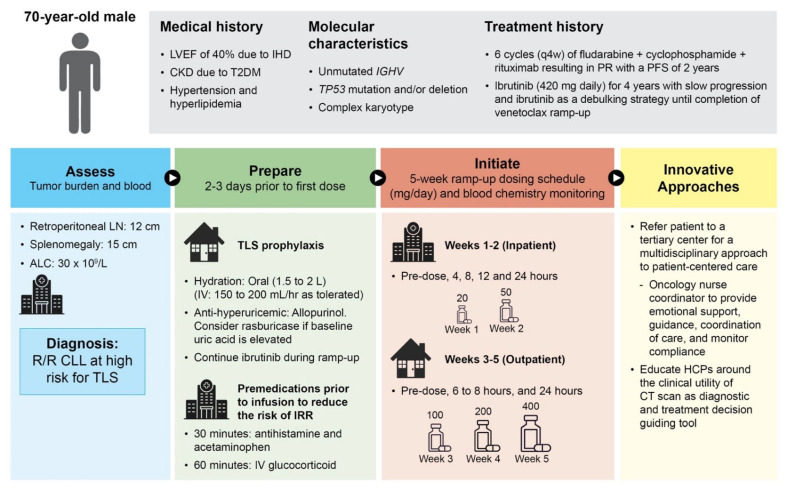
Patient case 1: High risk for tumor lysis syndrome (relapsed patient treated with continuous venetoclax). ALC, absolute lymphocyte count; CKD, chronic kidney disease; CLL, chronic lymphocytic leukemia; CT, computerized tomography; HCP, healthcare provider; IGHV, immunoglobulin heavy variable; IHD, ischemic heart disease; IV, intravenous; IRR, infusion-related reaction; L, liter; LN, lymph node; LVEF, left ventricular ejection fraction; mg, milligram; PFS, progression-free survival; PR, partial response; q4w, every 4 weeks; R/R, relapsed/refractory; T2DM, type 2 diabetes mellitus; TLS, tumor lysis syndrome; TP53, tumor protein 53.
